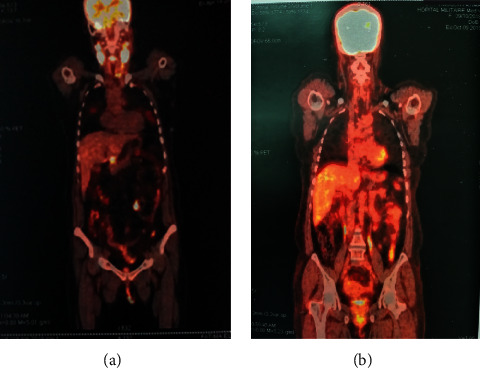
(a) PET/CT at diagnosis showed hyperpathological lymph node metabolism over and under the diaphragmatic involvement. (b) PET/CT after treatment showed a complete metabolic remission.
